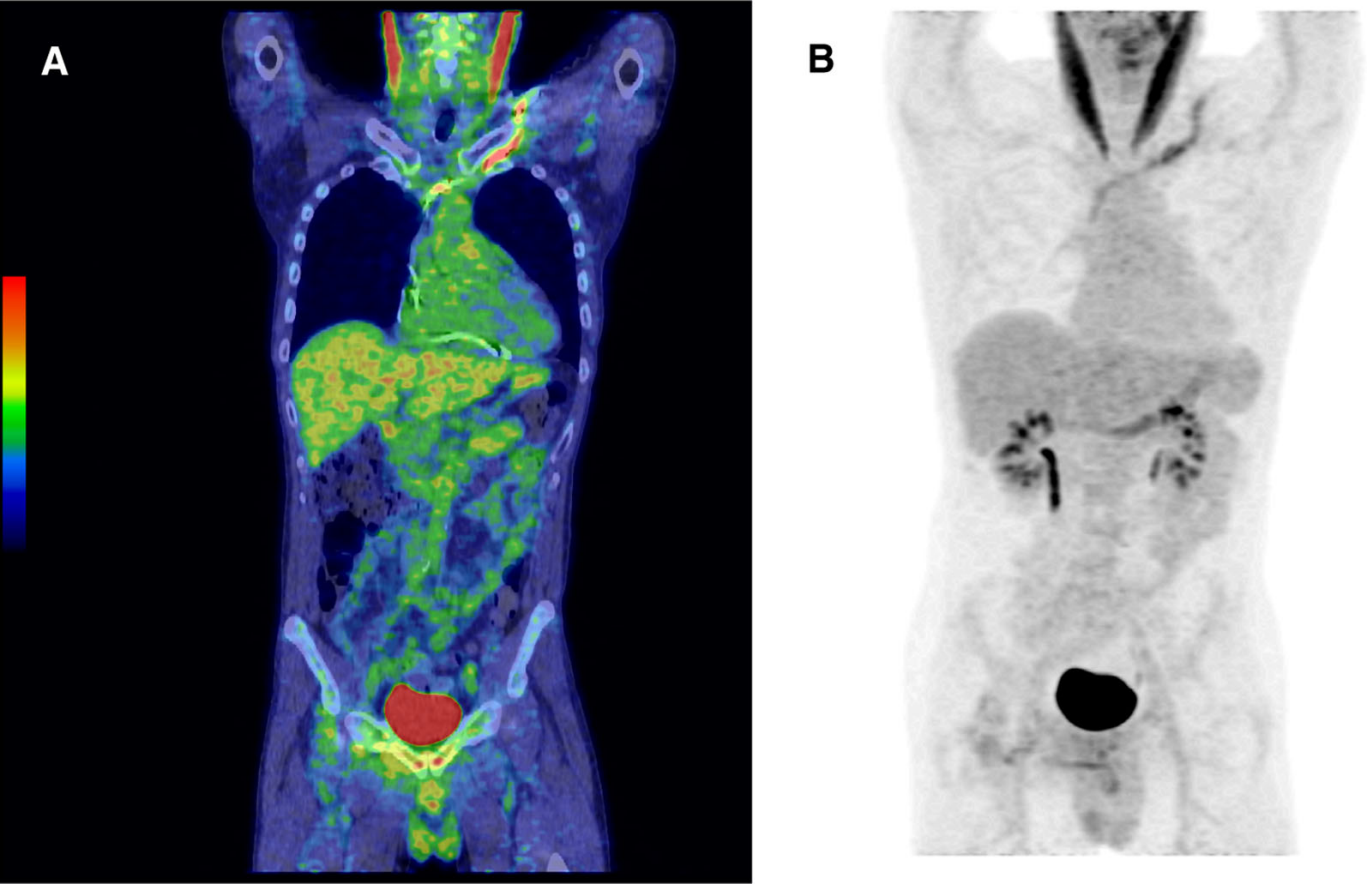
Patient #23 had fever of unknown origin, but no symptom in CIED area. The generator had been changed 1.5 years before. ^18^F-FDG-PET/CT showed uptake in lead, SUV_max_ 5.2 (Panel A and B). Bacterial culture from removed CIED system yielded *Staphylococcus epidermidis*.
